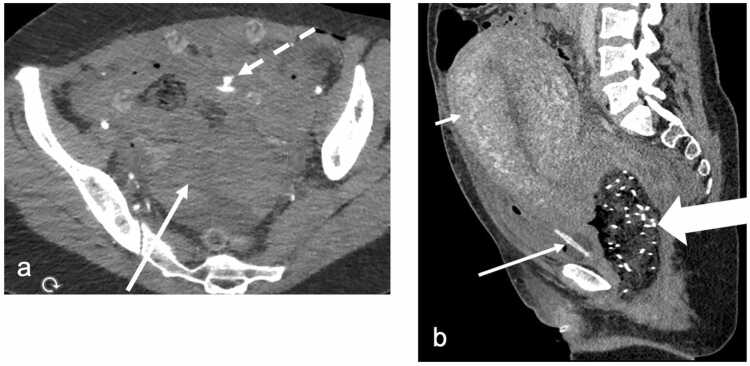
a) Arterial phase CT in patient with postpartum haemorrhage. CT demonstrates large volume haemoperitoneum (long arrow) and active bleeding (dashed arrow). The patient was successfully treated with internal iliac embolization. DIAGNOSIS: postpartum haemorrhage. b) Post partum haemorrhage due to vaginal laceration treated with vaginal packing (block arrow). No active bleeding, packing is dry. Catheter in bladder (long arrow) and normal vascular myometrium (short arrow) noted. DIAGNOSIS: postpartum haemorrhage.
